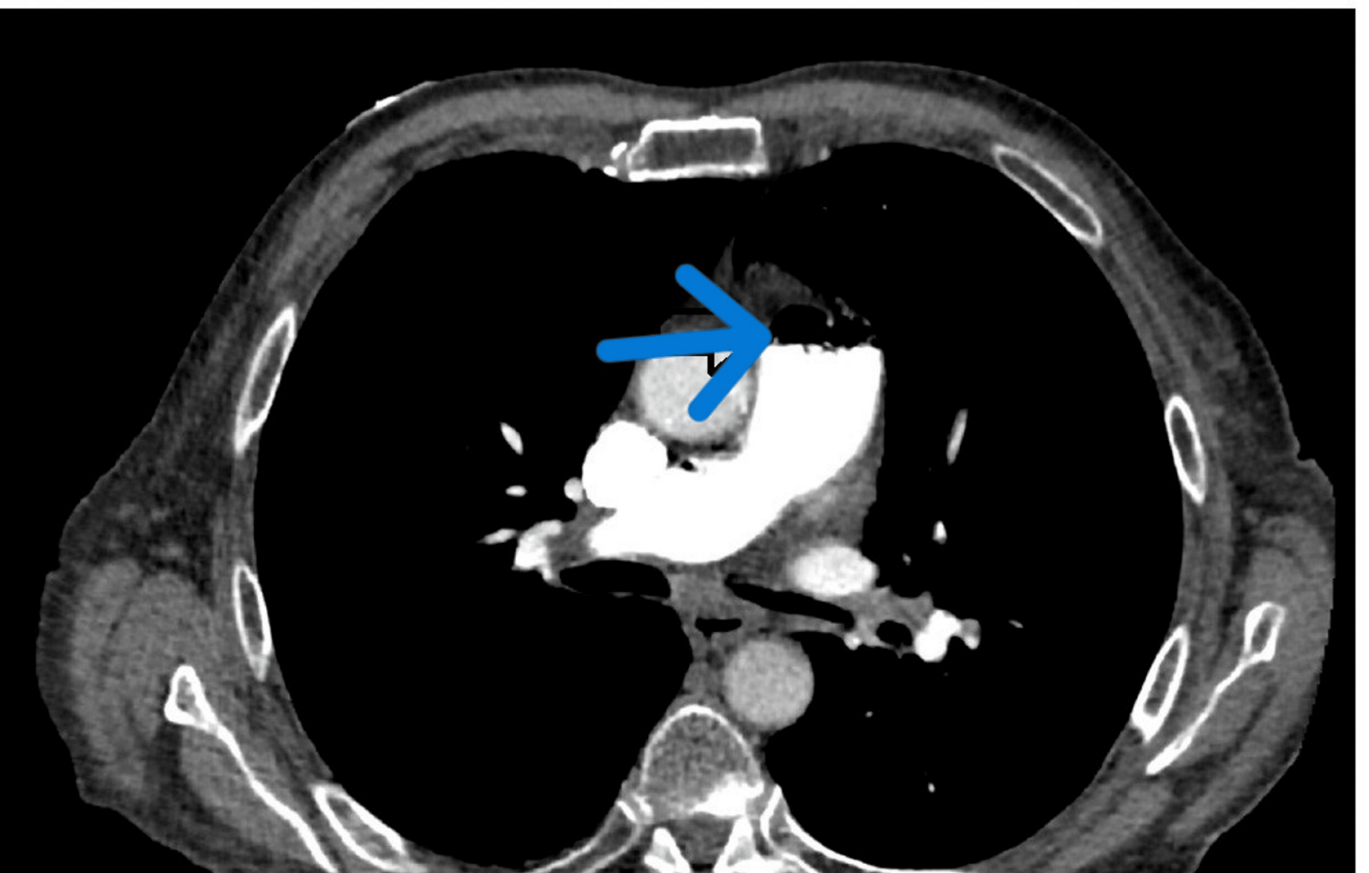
Axial view of a computed tomography (CT) scan of the chest, showing a small to moderate volume of air embolism (indicated by the arrow) within the main pulmonary artery and right ventricle.
